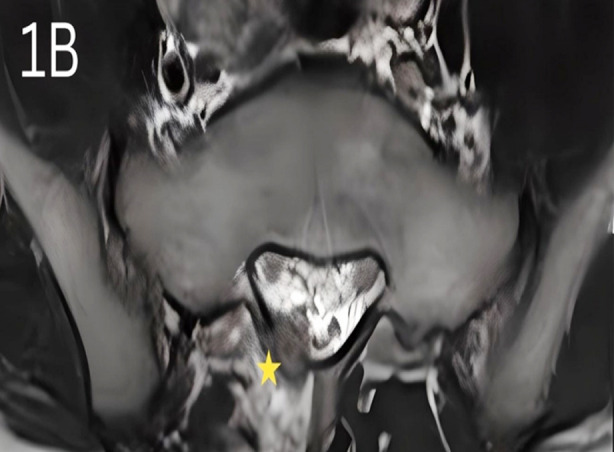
Contrast-enhanced MRI of Lumbosacral spine showing an enhancing lesion marked by a yellow star.
